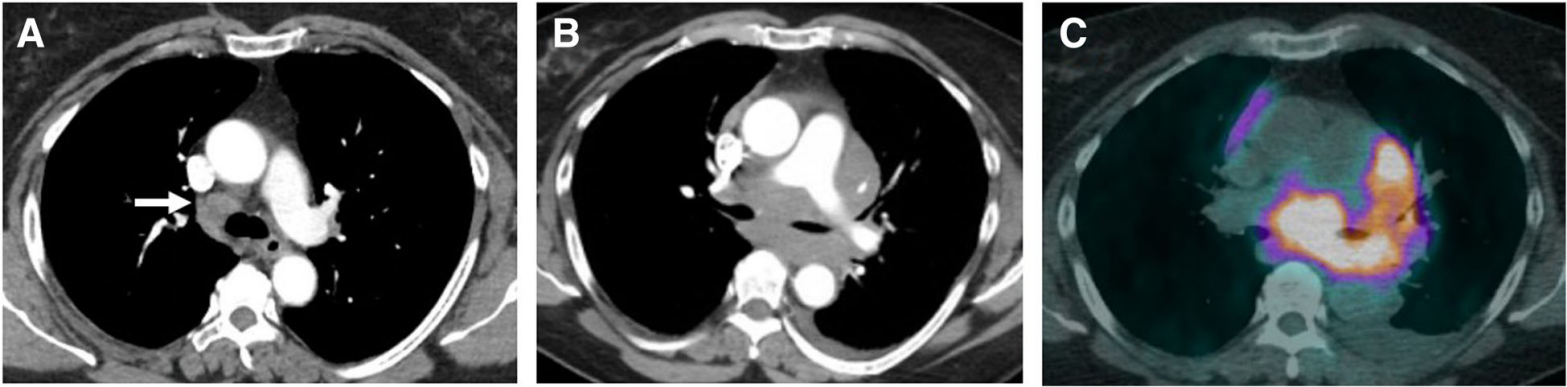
A 60-year-old woman with grade I follicular lymphoma on rituxan, with new onset shortness of breath and atrial fibrillation. a Axial CT image of the chest acquired during arterial phase 1 year before onset of new symptoms shows mildly prominent mediastinal lymph nodes (arrow). b Axial CT image acquired during arterial phase at time of symptoms shows a large amorphous mediastinal mass surrounding the distal trachea and the pulmonary artery. c Axial PET/CT fused image of the chest acquired at time of symptoms shows avid FDG uptake of the mass, with SUVmax 20.1. The lesion was biopsied and showed grade III follicular lymphoma
